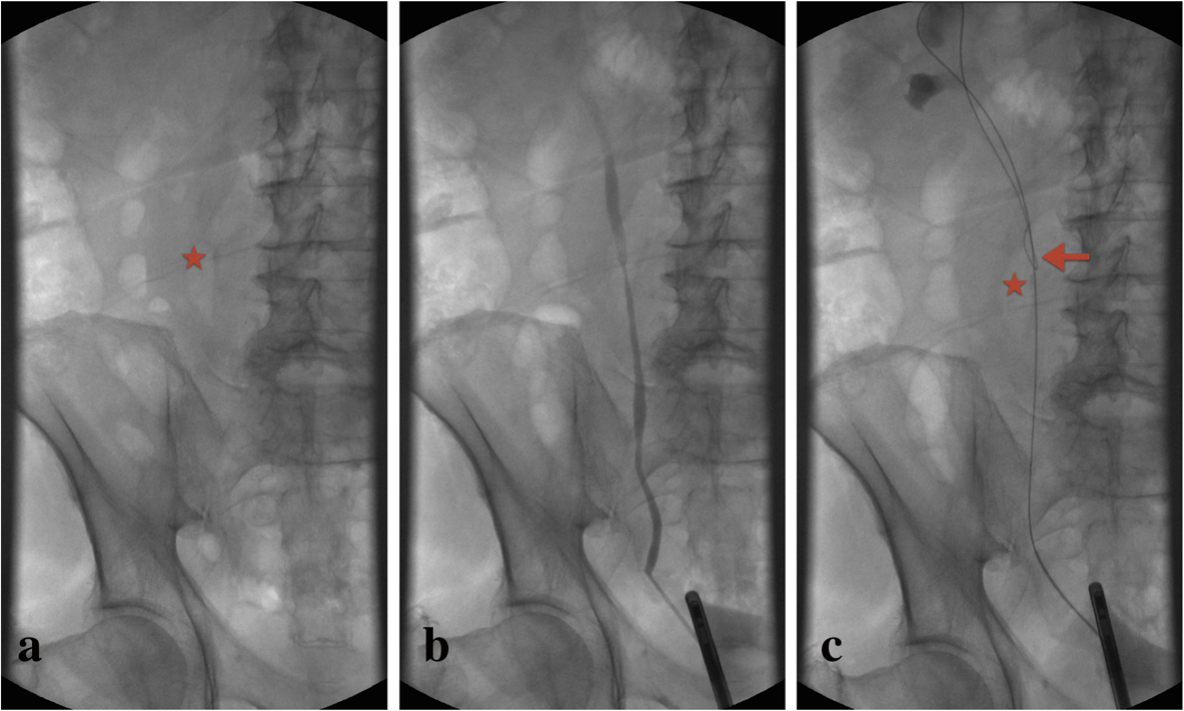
Step-by-step description of the “Guidewire-Coil-Technique” based on the example of a mid ureteric stone. a: Plain x-ray; b: Retrograde pyelography; c: Correctly placed coil of the guidewire. *Asterisk = Ureteral calculus, arrow = Reverted guidewire acting as a counterfort*
